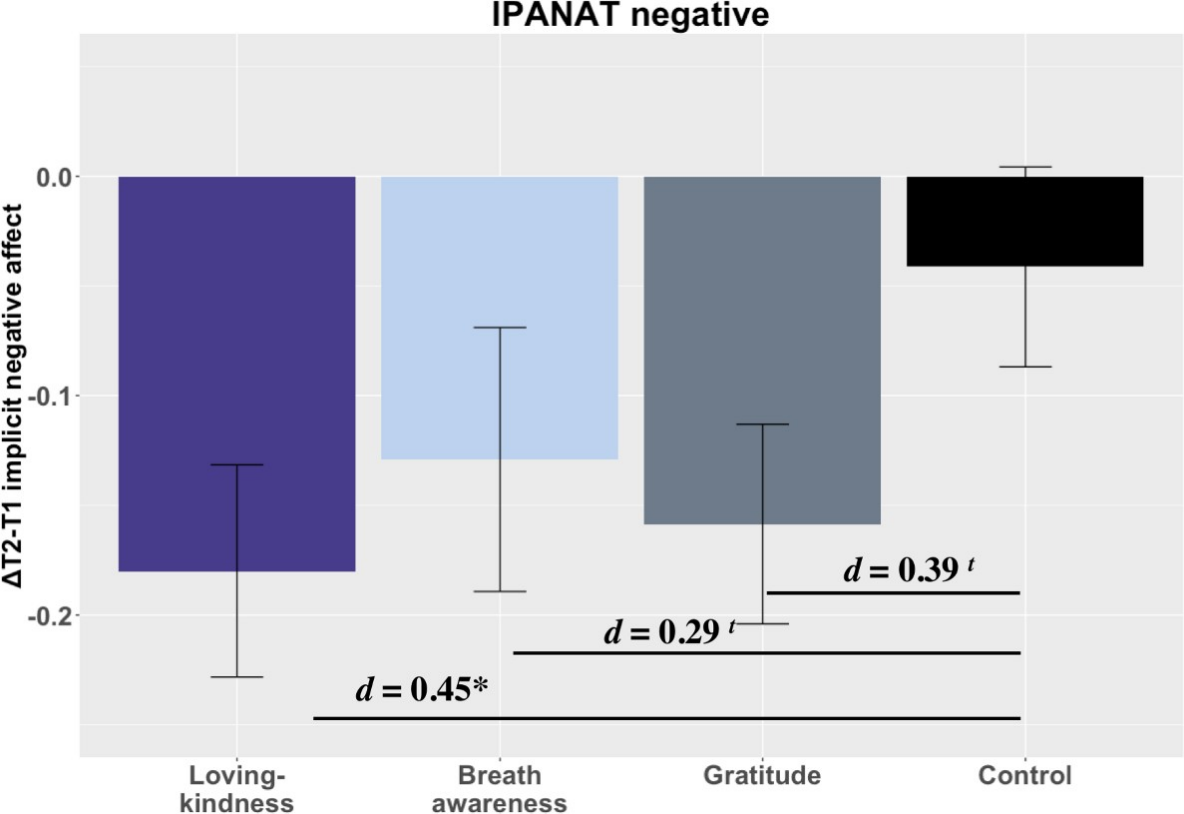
Training changes in negative affect. Notes: * = *p* < .001, * = p* < .01,  = p <* .05, *t = p* < .10. Loving-kindness = LK; Breath awareness = BA; Gratitude = GT; Control = CT.
